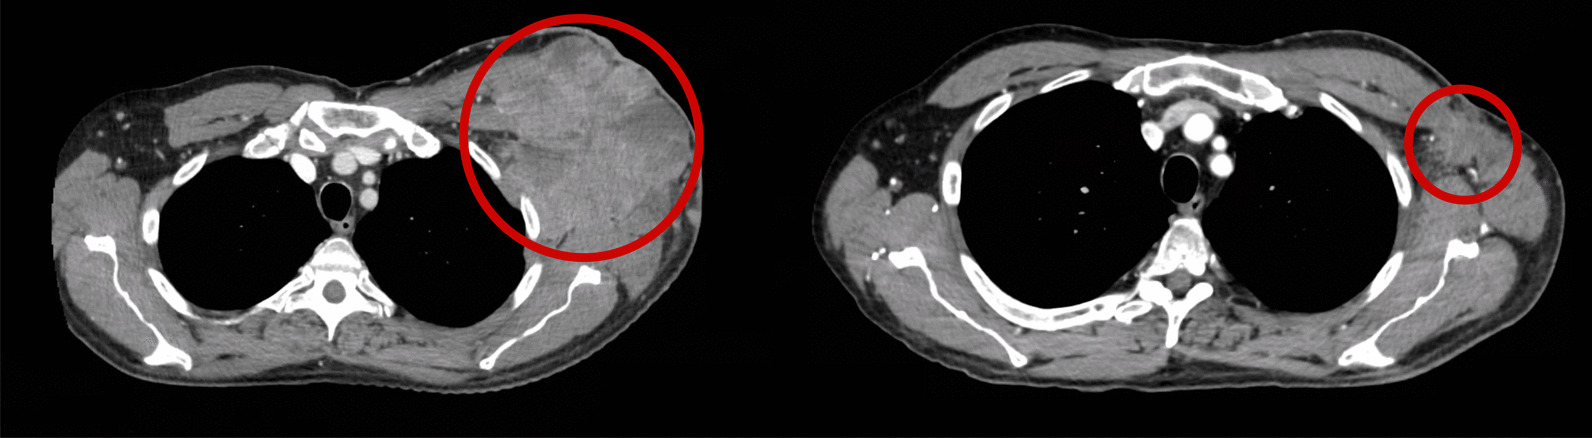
Axial computed tomography scan of chest for patient 2 before treatment (left) and axial computed tomography scan of patient 2’s chest after treatment with radiation, anti-PD-1, and high-dose IL-2 (right), demonstrating substantial reduction in tumor volume
